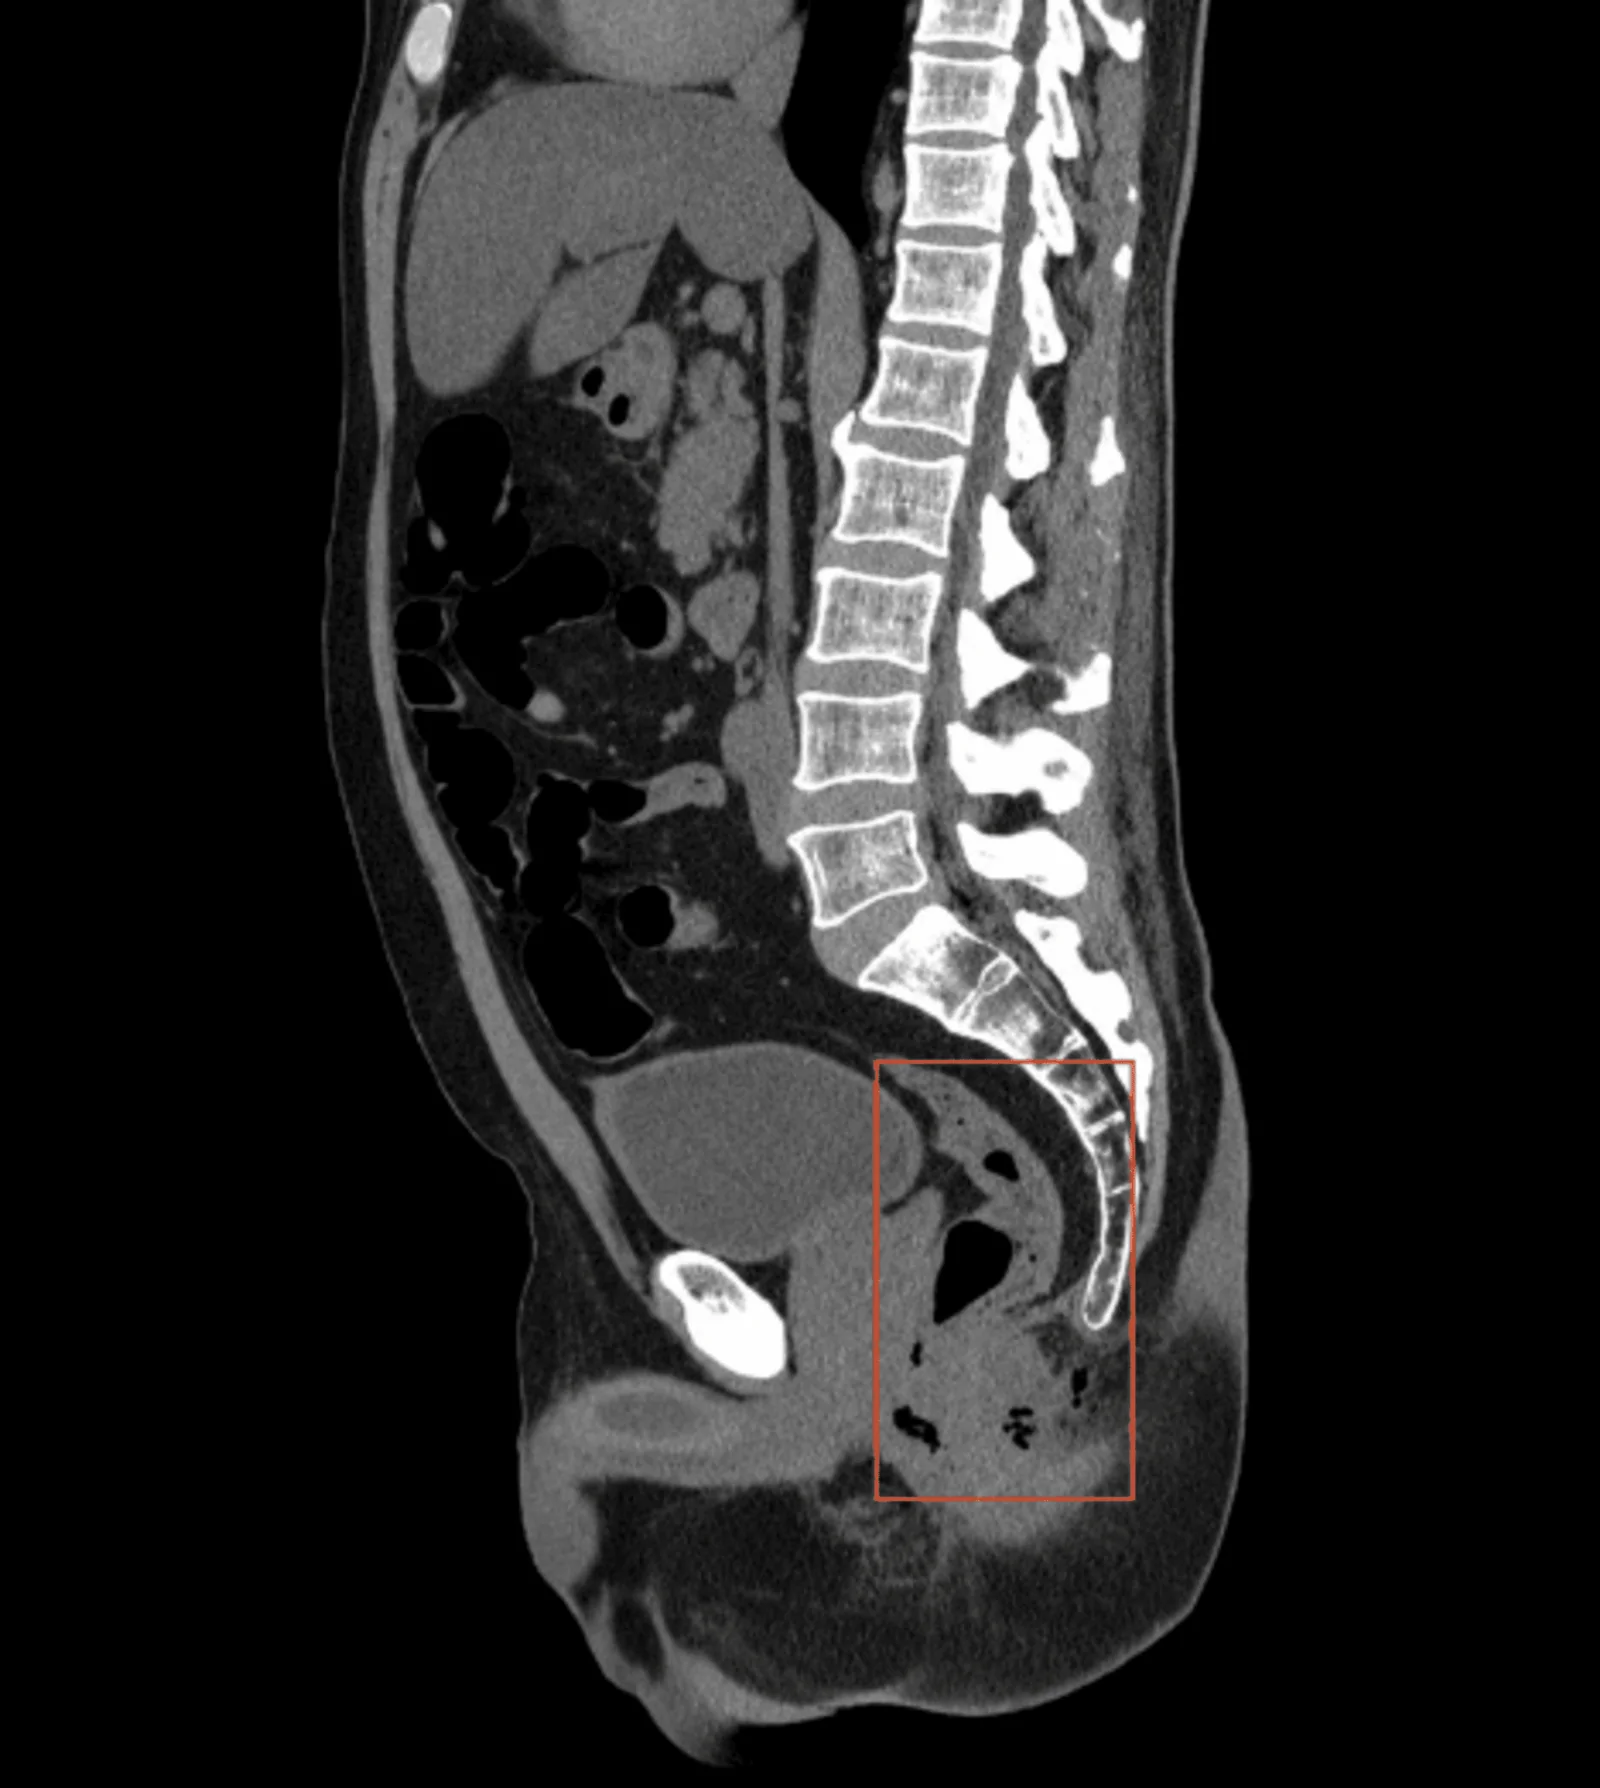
Sagittal computed tomography of the abdomen and pelvis. Sagittal computed tomography (CT) image of the abdomen and pelvis showing soft tissue gas extending into the perineum (red box), concerning for Fournier gangrene.
